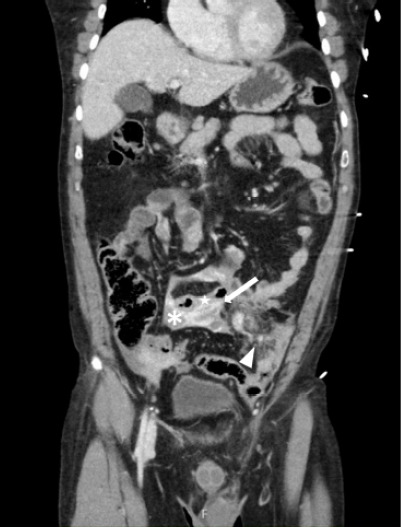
Abdominal computed tomography (coronal image) with active extravasation of contrast (arrow), free fluid (asterisk), bowel wall edema (star) and mesenteric fat stranding (arrowhead) in the lower abdomen, suggestive of mesenteric injury.
